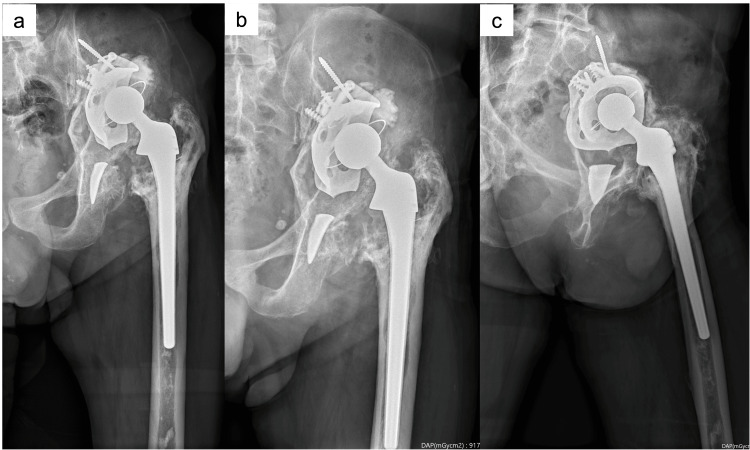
Radiograph findings (2022 and 2026) a: In 2022, eight years after the fracture of the distal flange, imaging showed an acceptable cage inclination angle and stability of the cemented cup. The fixation screws showed no signs of loosening. b, c: In 2026, 32 years after the first total revision hip arthroplasty, the Lord femoral stem remained stable, with no measurable subsidence or progressive radiolucent lines.
